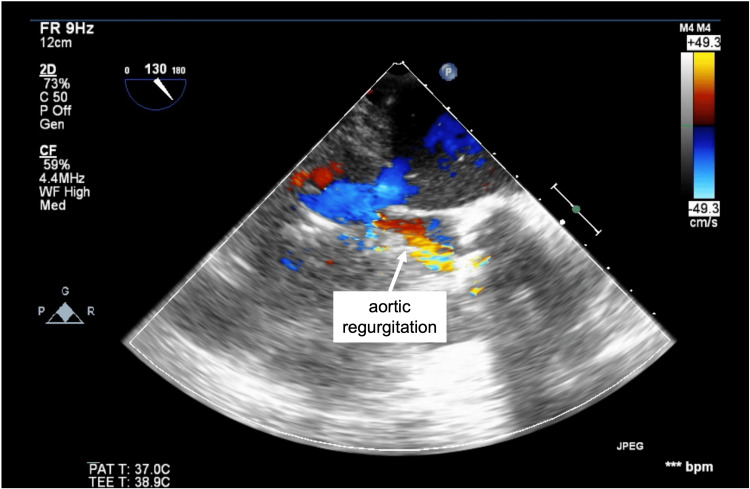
Acute moderate aortic regurgitation observed after transesophageal echocardiography insertion (case 1)
